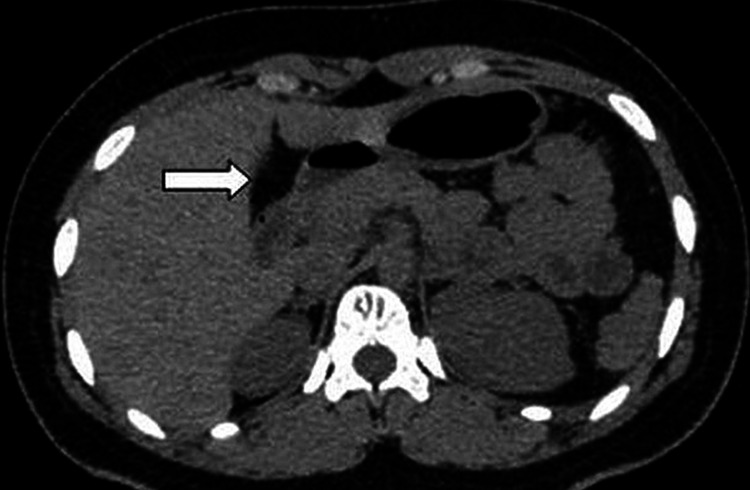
Axial non-contrast CT showing fat in the gallbladder fossa (arrow) with non-visualization of the gallbladder. CT - Computed Tomography.
